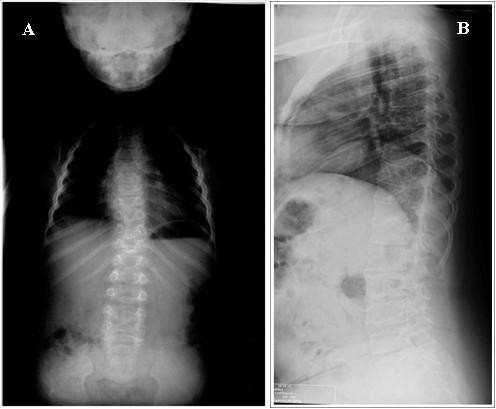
Radiographs of patient 1. A. 6 years. B. 28 years. Note generalized platyspondyly, thoracolumbar scoliosis.
